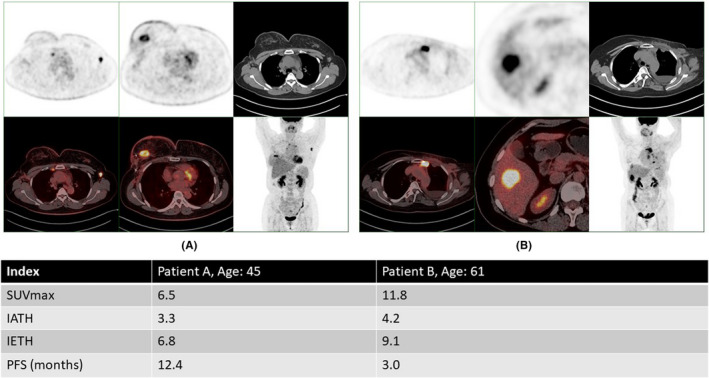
Analysis examples: Patient A was a 45‐year‐old female mTNBC patient. The following heterogeneity parameters of her metastatic lesions were obtained: SUVmax 6.5, IATH 3.3, and IETH 6.8 with a PFS of 12.4 months. Patient B was a 61‐year‐old female mTNBC patient. The following heterogeneity parameters of her metastatic lesions were obtained: SUVmax 11.8, IATH 4.2, and IETH 9.1 with a PFS of 3.0 months
